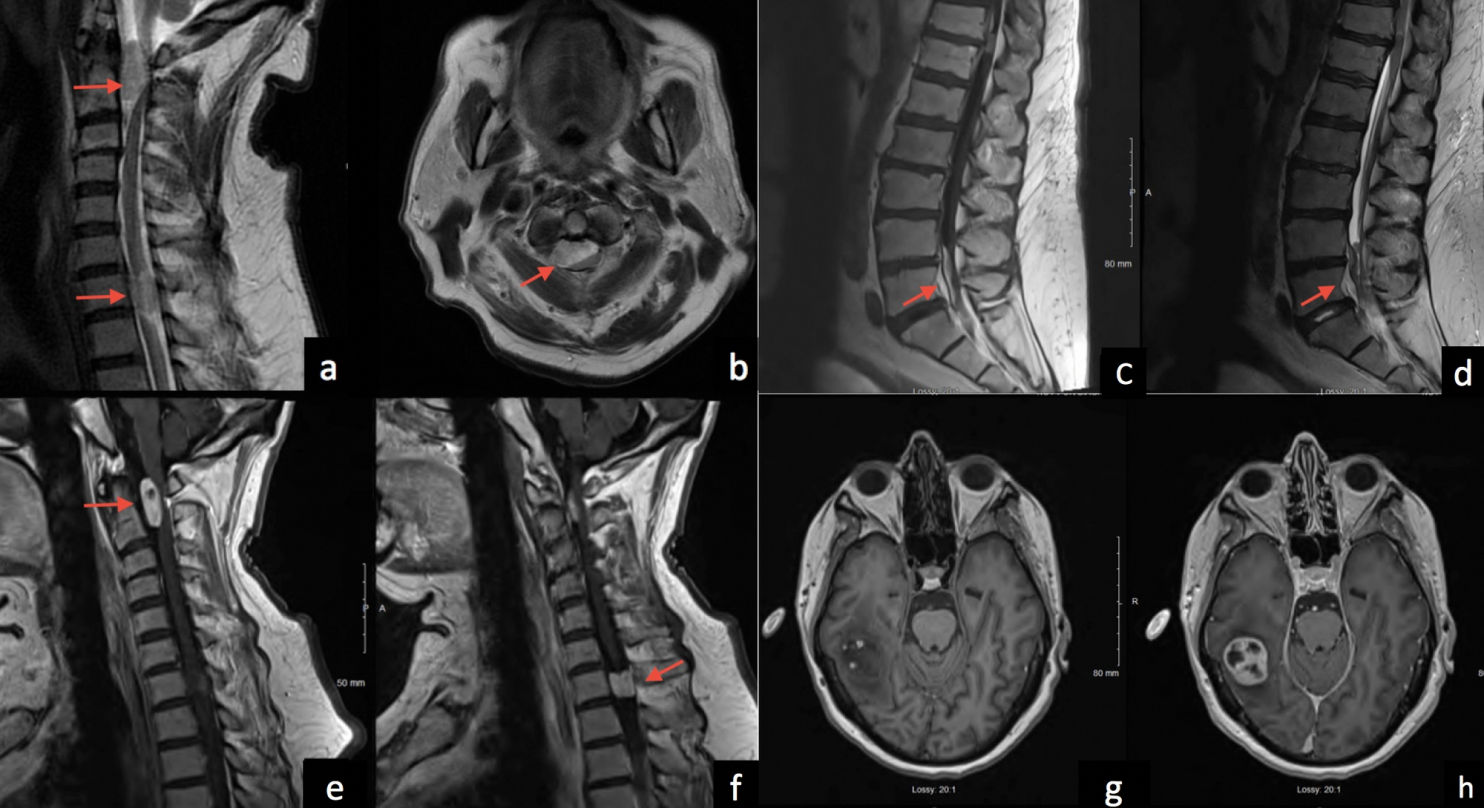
Magnetic resonance imaging of the cervical and lumbar spine and brain. T2-weighted magnetic resonance imaging (MRI) of the cervical spine in sagittal (a) and axial (b) planes, the latter at the C2 level, demonstrating intradural extramedullary lesions with compression of the spinal cord. The lesions are seen to brightly enhance on T1-weighted sagittal images with intravenous contrast (e-f). Sagittal T1 contrast-enhanced (c) and T2-weighted (d) MRI of the lumbar spine showing intradural extramedullary masses at the conus medullaris and L4-S1 levels. Axial MRI of the brain precontrast (g) and postcontrast (h) demonstrates an approximately 3 cm enhancing mass in the posterior right temporal region with perilesional edema.
